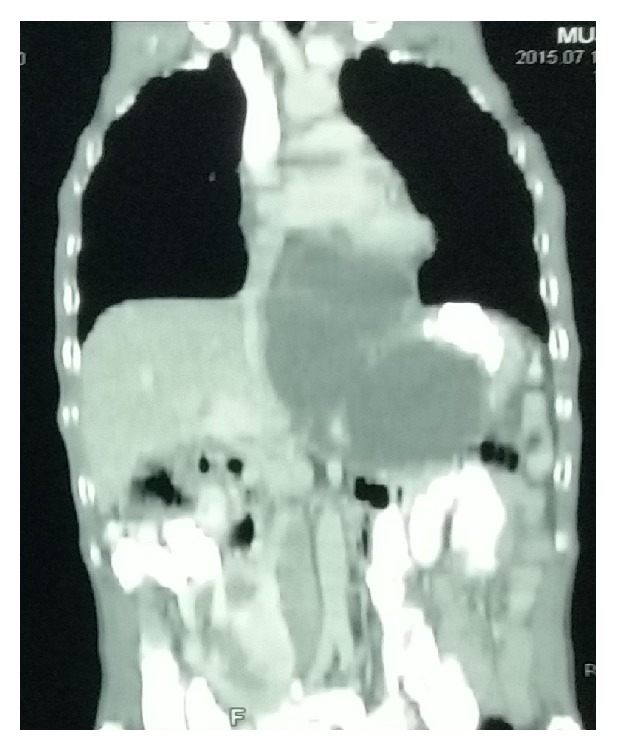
CT chest coronal view showing mediastinal pseudocyst of size 8 cm.
